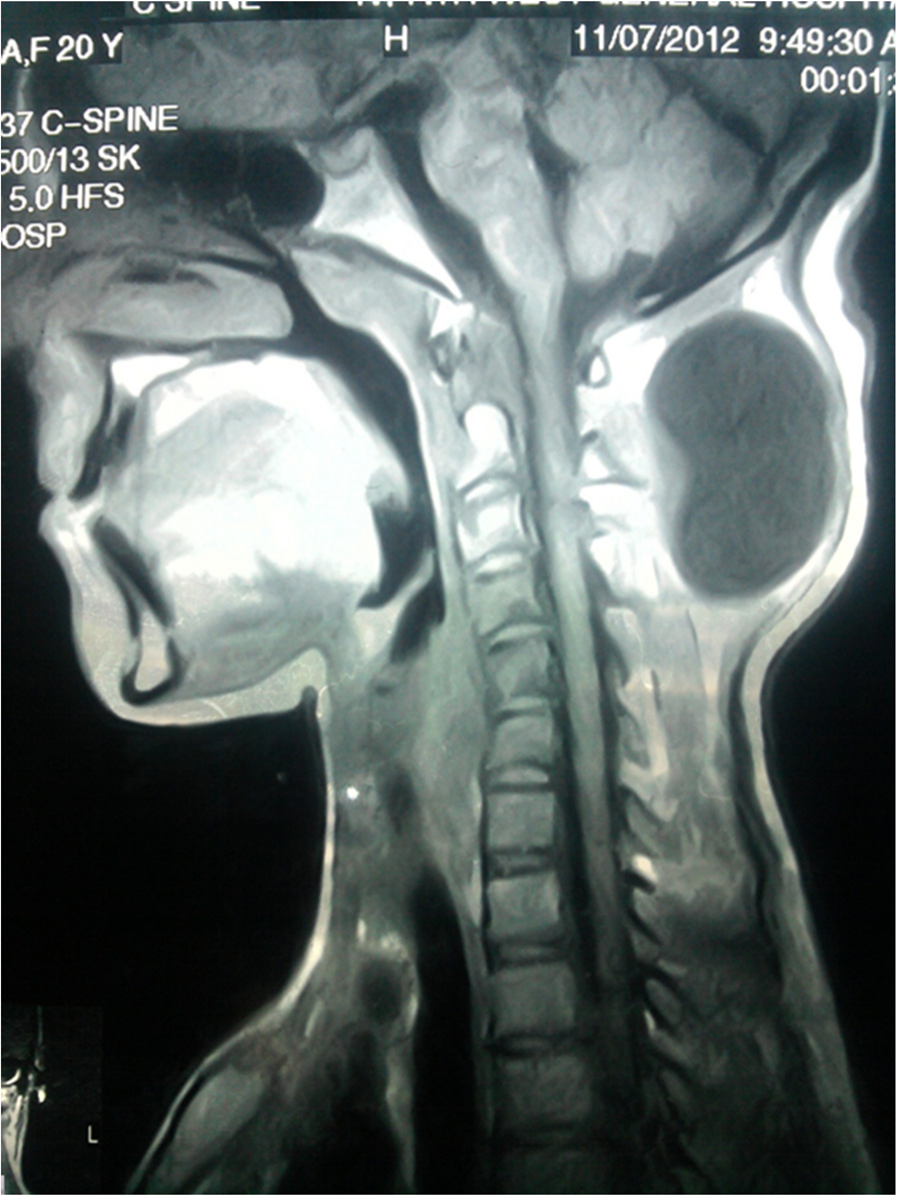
T1 weighted MRI without gadoliniumcontrast shows a hypo-intense cystic mass (6.5x6.1 cm) in the posterior soft tissue at the craniovertebral junction.
